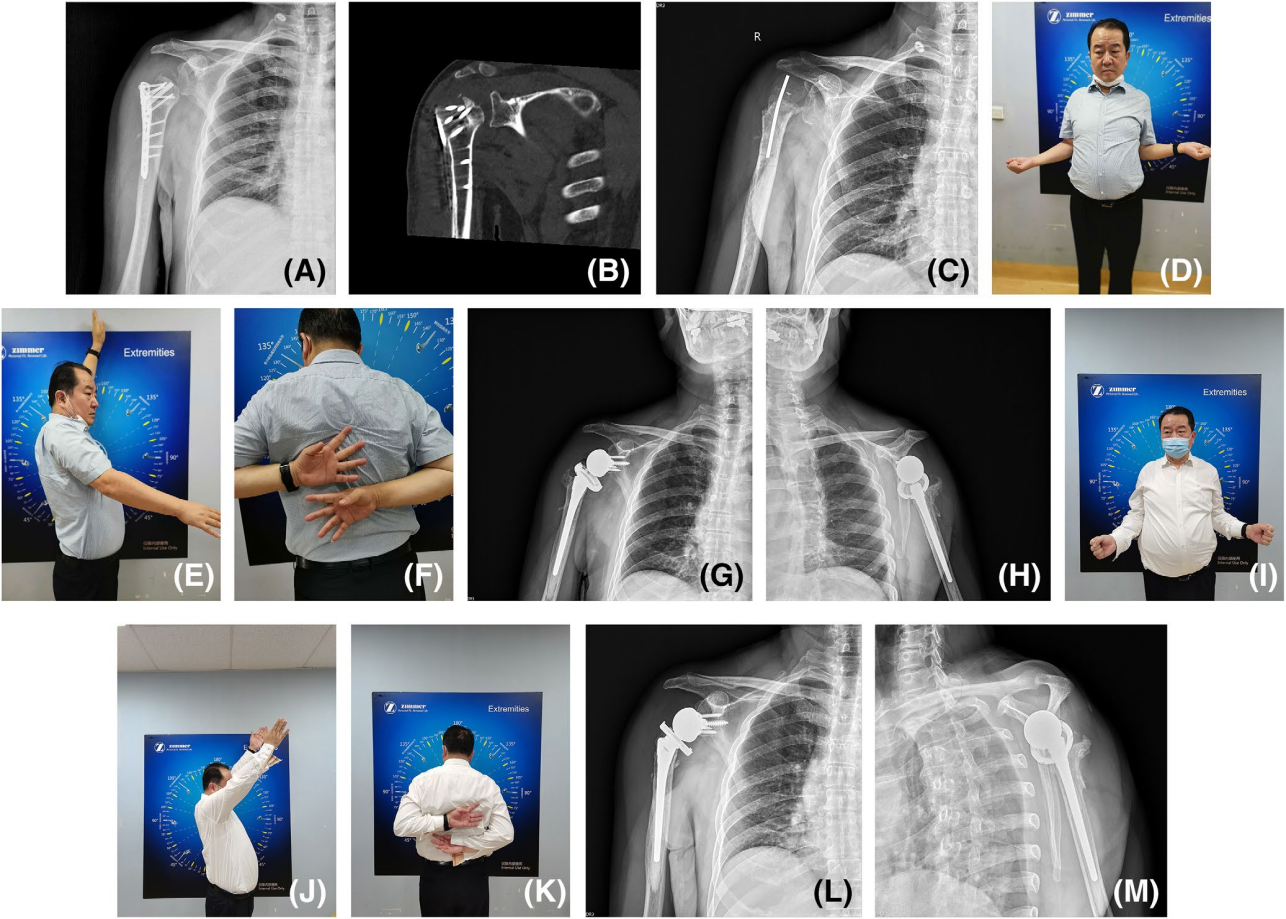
Representative case of a 53‐year‐old male with post‐ORIF infection and proximal humeral bone loss. (A, B) Radiographic examinations showed a severe bone defect of the proximal humerus. (C) A thorough debridement and antibiotic cement spacer were introduced to the shoulder joint. (D–F) Functional limitations prior to salvage RSA. (G, H) A two‐stage revision RSA was performed for the patient. The postoperative X‐rays showed that the prosthesis position was satisfactory after RSA. (I–K) 6 months after surgery, the forward elevation was 140°; external rotation was 30°; IR was T12 level. VAS score was 0; The ASES score was 88; Constant‐Murley score was 89. (L, M) 14 months after surgery, the X‐rays showed no signs of loosening.
